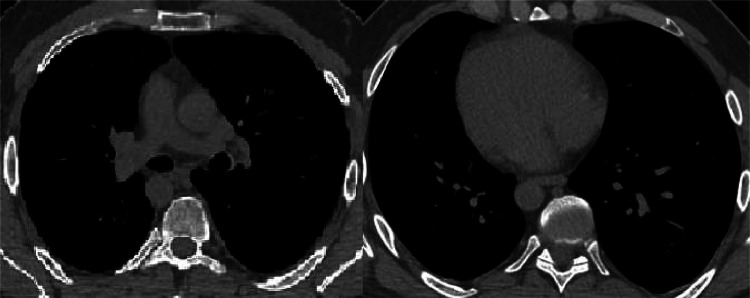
An axial CT image of the chest, without contrast medium confirmed the condition of dextrocardia, and the apex of the heart is on the right.
